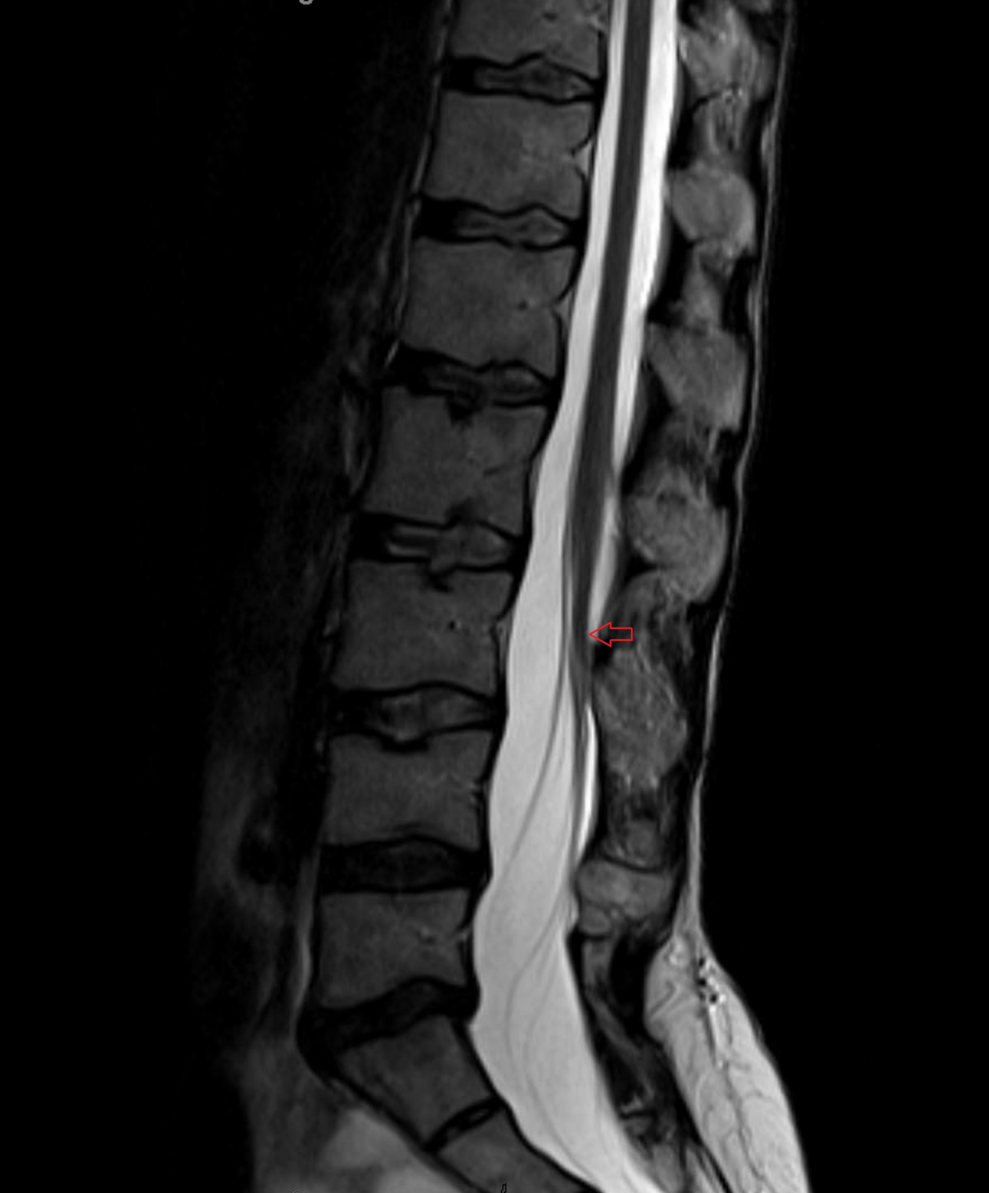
Sagittal T2 magnetic resonance imaging scan in the supine position showing a low-lying conus at the level of the mid-body of the L3 vertebral body (red arrow)
